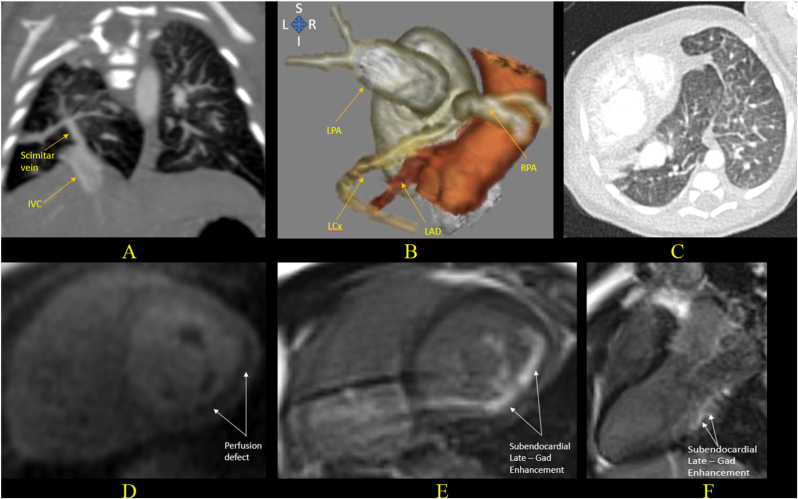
Images of a 6-day-old girl with SS. (A) CT image in the coronal plane shows anomalous drainage of the right lung via a scimitar vein into the hepatic IVC. (B) 3D volume rendered image shows a hypoplastic RPA with measurement 3 mm, compared to the LPA that measured 6.7 mm. The LCx coronary artery arises from the hypoplastic RPA while the LAD arises normally from aorta. (C) CT image in axial plane shows dextroposition of the heart and horseshoe segment of right lung passing between the aorta and heart, appearing confluent with the left lung. This patient had catheter embolization of an aortopulmonary collateral and surgical reimplantation of the LCx coronary artery in infancy. (D, E, F) CMR stress perfusion and late gadolinium enhancement (LGE) images after the surgery at the age of 10 years show a persistent perfusion defect and subendocardial LGE in the infero-lateral wall, basal and mid portion of LV, consistent with the infarction of the LCx territory. SS: Scimitar Syndrome, CT: Computed Tomography, IVC: Inferior Vena Cava, RPA: Right Pulmonary Artery, LPA: Left Pulmonary Artery, LCx: Left Circumflex Artery, LAD: Left Anterior Descending Artery, CMR: Cardiac Magnetic Resonance, LGE: Late Gadolinium Enhancement, LV: Left Ventricle.
